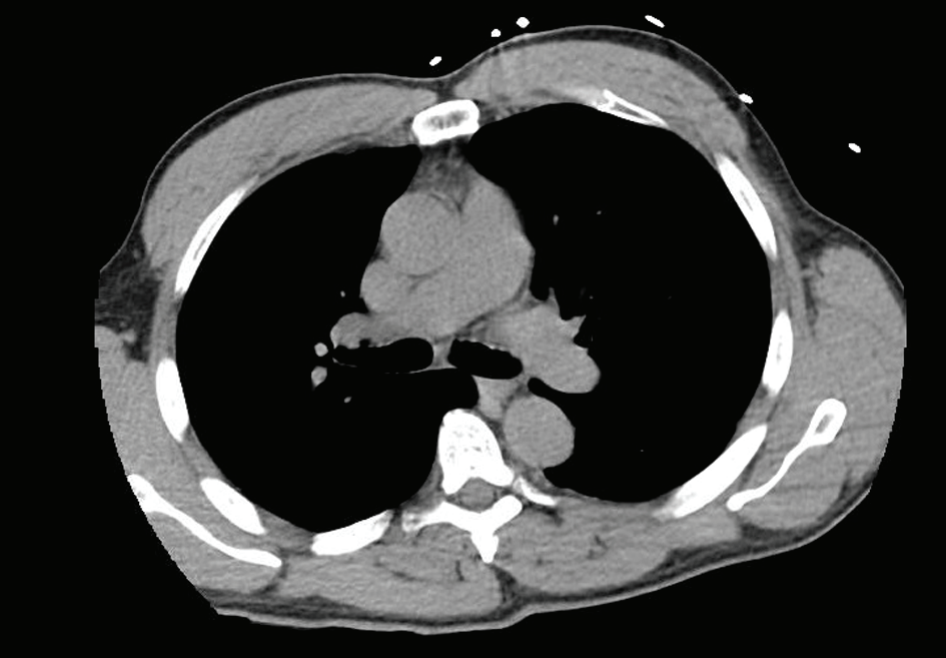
CT angiogram conducted showing absence of pulmonary embolism.
